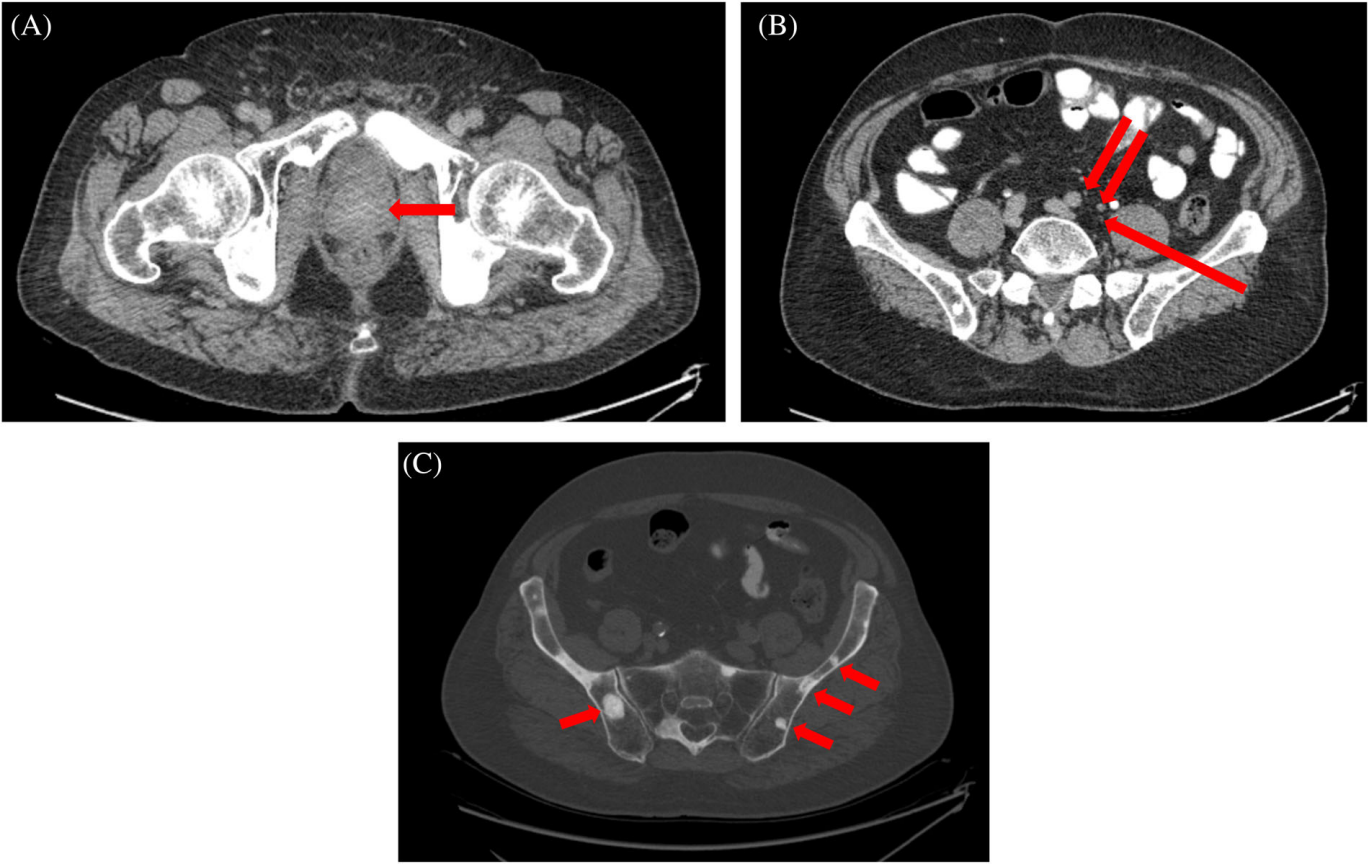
(A) Pelvic computed tomography scan showing prostate (arrow), (B) para‐aortic lymphadenopathies (arrows), and (C) metastatic pelvic bone lesions (arrows).
